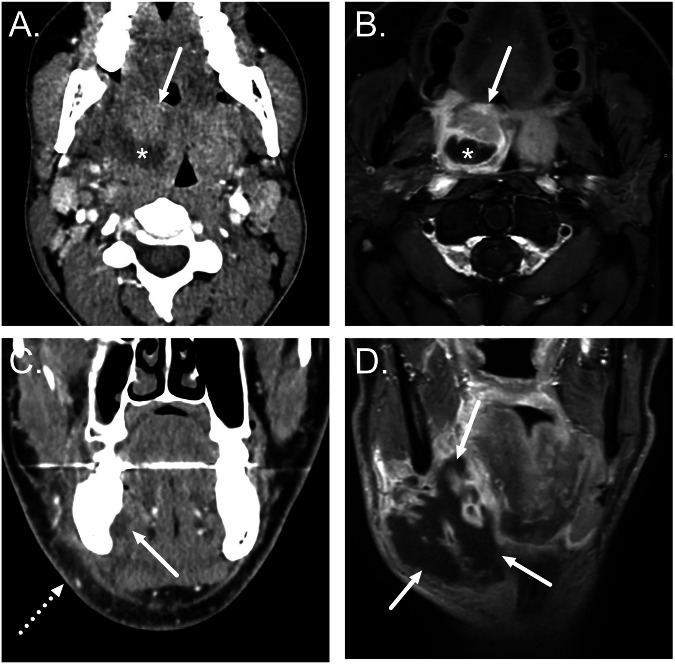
A, B Examples of peritonsillar abscesses. A Axial CT image shows a hypodense collection (asterisk) behind the right palatine tonsil (arrow). B Axial fat-suppressed post-contrast T1-weighted image shows a non-enhancing hypointense collection (asterisk) behind the right palatine tonsil (arrow). C, D Examples of odontogenic neck abscesses. C A coronal CT image shows a hypodense collection (arrow) medial to the mandible, consistent with a subperiosteal abscess. Note the subcutaneous fat stranding (dotted arrow). D A coronal fat-suppressed post-contrast T1-weighted image shows a large multilocular abscess extending from the submandibular space to the anterior cervical space
